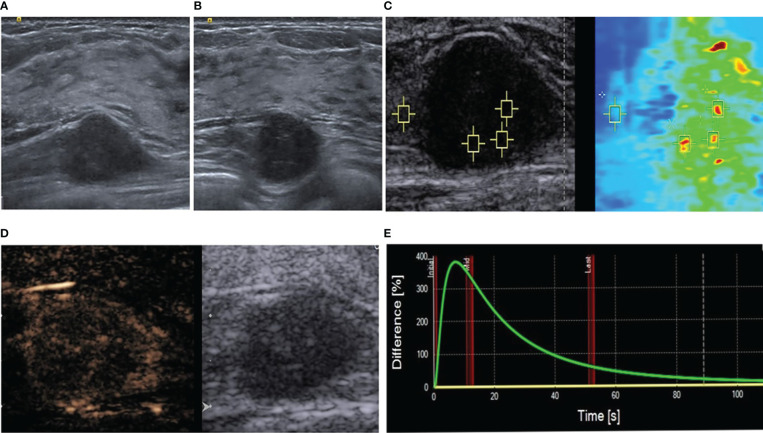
Multimodal ultrasonographic assessment of the mass. (A) Long-axis ultrasound showing a hypoechoic mass located deep in the pectoralis major muscle; it was deep in the gland and the upper right breast. (B) The adjacent glands appear blurred. (C) Virtual touch tissue imaging and quantification mode. The depth is 2.4 cm, median speed 6.06 m/s, and mean speed 6.18 m/s. The surrounding muscular layer is 2.72 m/s. (D) Qualitative contrast-enhanced ultrasound results. (E) Time-intensity curve.
